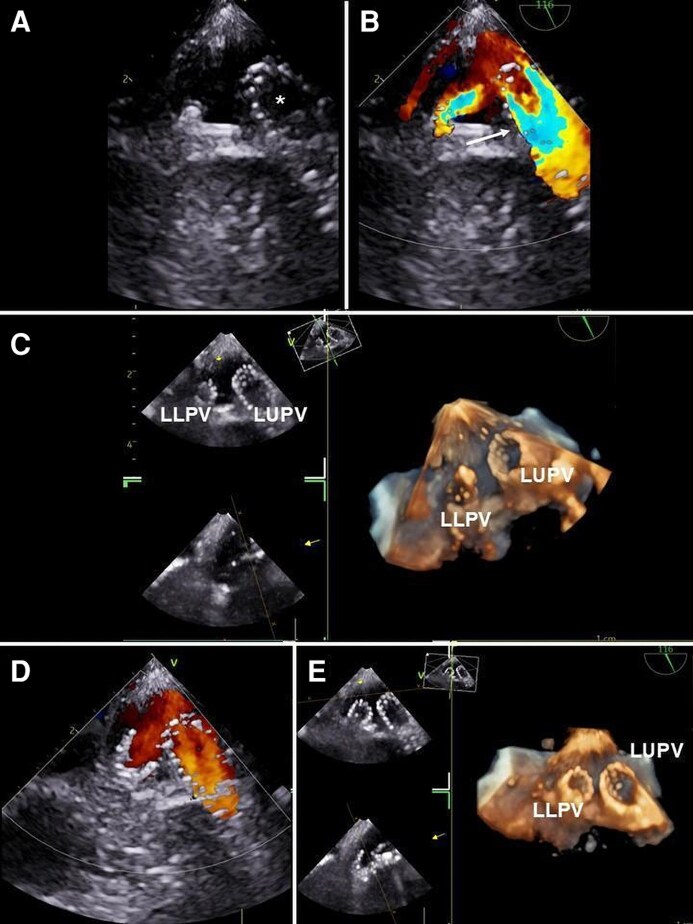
After balloon dilatation or stent placement, TOE is essential not only for assessing procedural success (defined as a mean pulmonary vein gradient <2 mmHg and residual stenosis <20%) but also for detecting residual stenosis. In addition, TOE allows evaluation of the immediate haemodynamic effects, including biventricular function and pulmonary artery pressure, and the identification of potential complications such as pericardial effusion or residual interatrial septal defect. (*A*) Stent implanted into the LUPV (asterisk). (*B*) 2D colour Doppler of the LUPV after stent implantation showing aliasing within a dilated vein (white arrow). (*C*) 3D real-time view of stent implanted in the LUPV and stent dilatation in the LLPV. (*D*) 2D colour Doppler of the left PVs post-stents implantation showing laminar flow across the vessels. (*E*) 3D en-face view of the stents implanted in the left PVs. LLPV: left lower pulmonary vein; LUPV: left upper pulmonary vein; PV: pulmonary vein; TOE: transesophageal echocardiography.
